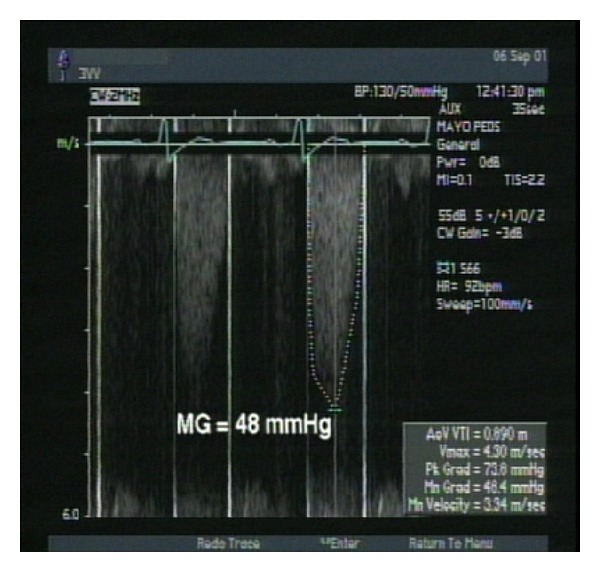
Continuous-wave Doppler recording from the preoperative transthoracic echocardiogram. This Doppler recording from the apical position demonstrates a mean gradient (MG) of 48 mmHg across the aortic valve prosthesis.
